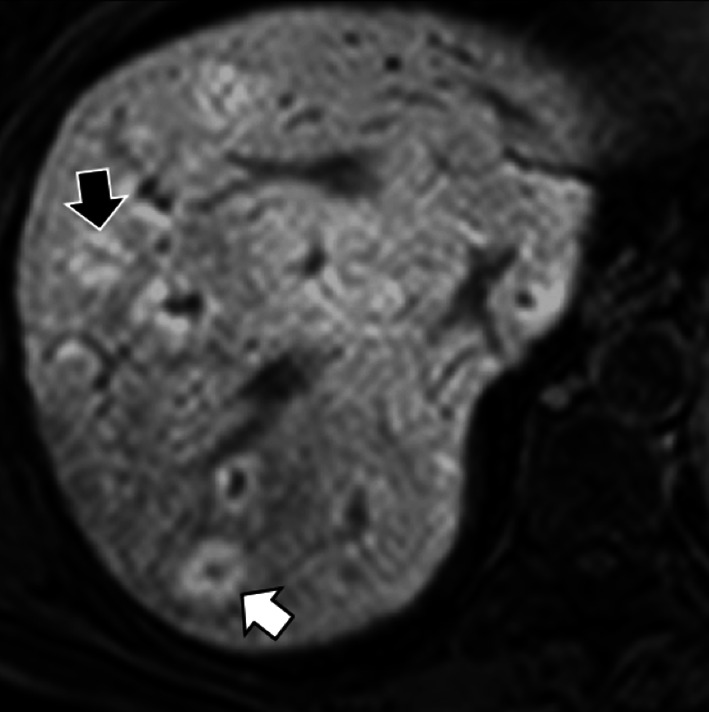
A 57-year-old woman with secondary sclerosing cholangitis and periportal hyperintensity on hepatobiliary phase. Gadoxetate disodium-enhanced MRI shows both thick tramline-like periportal hyperintensity (black arrow) and nodular-like periportal hyperintensity (white arrow)
